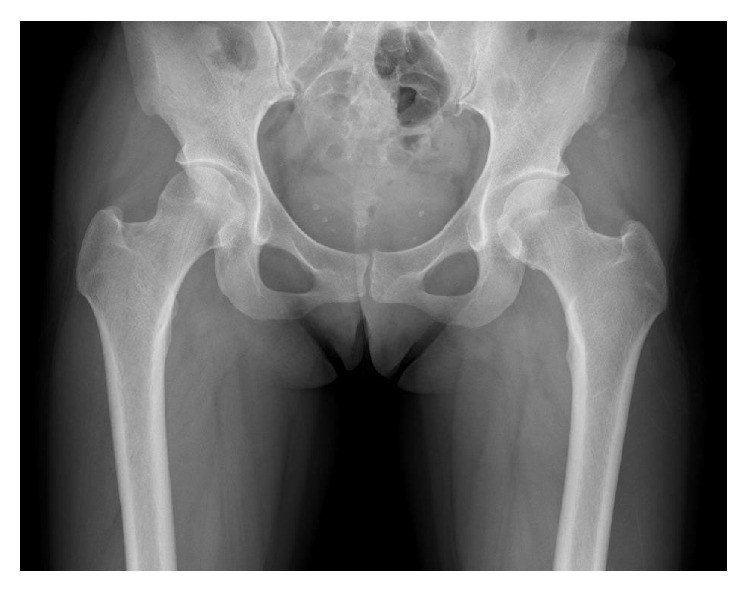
Anteroposterior X-ray of pelvis one week after symptom onset. No osteopenia.
